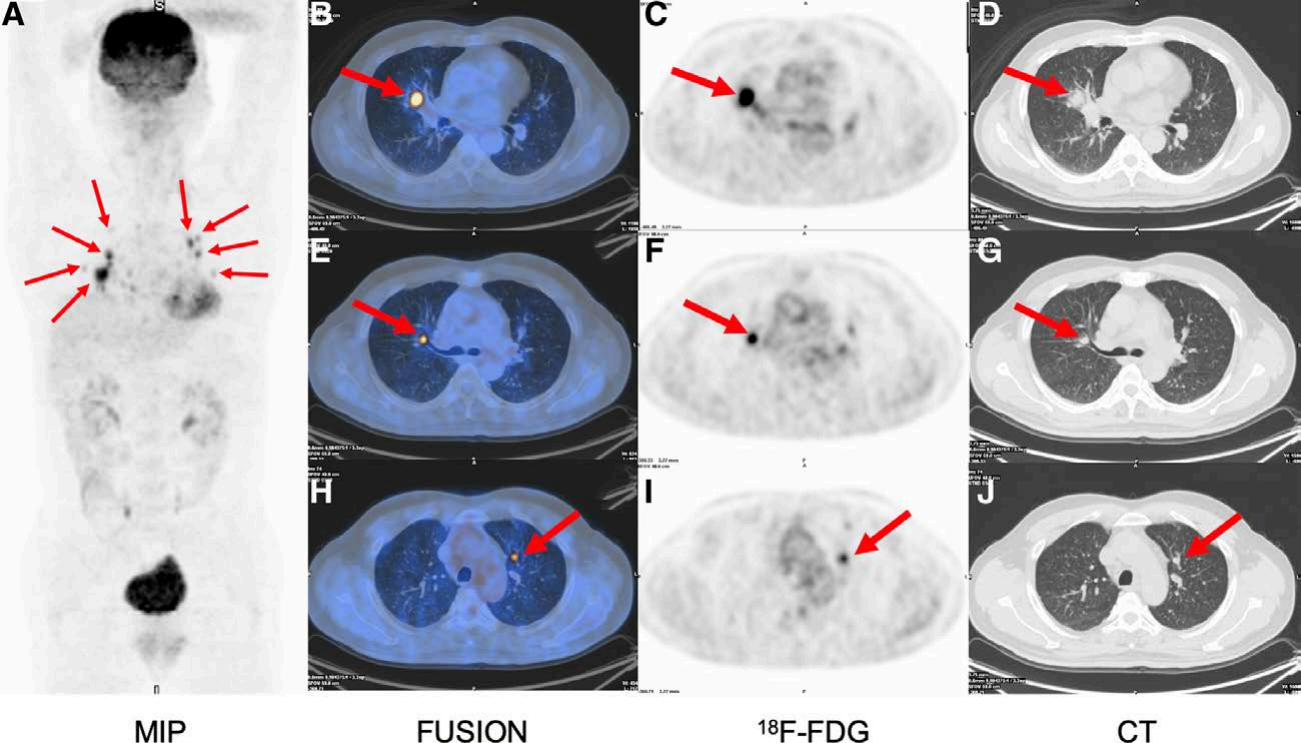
^18^F-FDG PET/CT. (A) ^18^F-FDG MIP showing multiple lung metastases with increased FDG uptake (red arrows). (B, E, H) Transaxial fused PET/CT. (C, F, I) Transaxial PET. (D, G, J) Transaxial CT. ^18^F-FDG = 2-deoxy-2-[^18^F]fluoro-D-glucose, MIP = maximum intensity projection.
